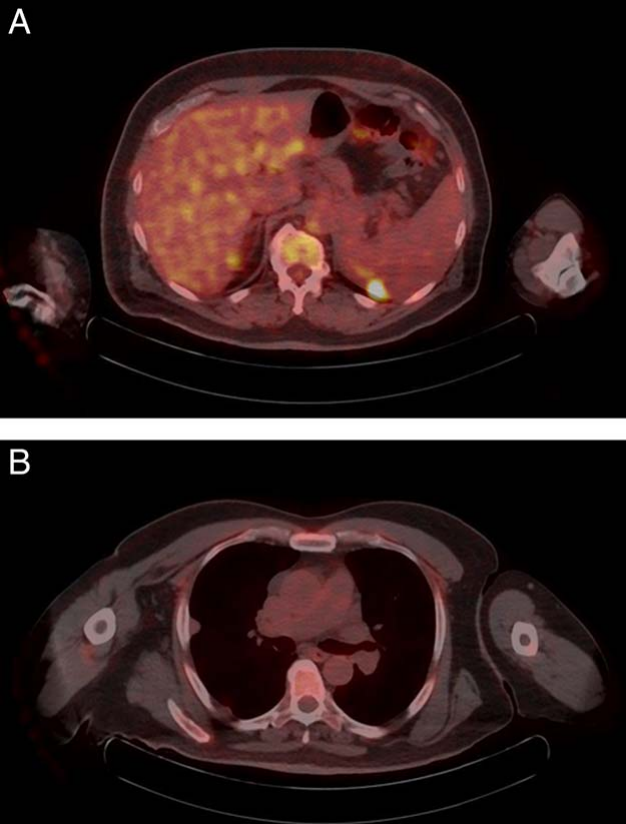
Positron emission tomography images at the time of diagnosis. (A) The mass exhibited minimal nonspecific heterogeneous fluorodeoxyglucose (FDG) activity and measured ~7×4.5 cm in its maximal axial dimensions. (B) The scan revealed multiple bilateral pulmonary nodules throughout both lung fields, showing variable degrees of FDG avidity, suggesting a metastatic process.
